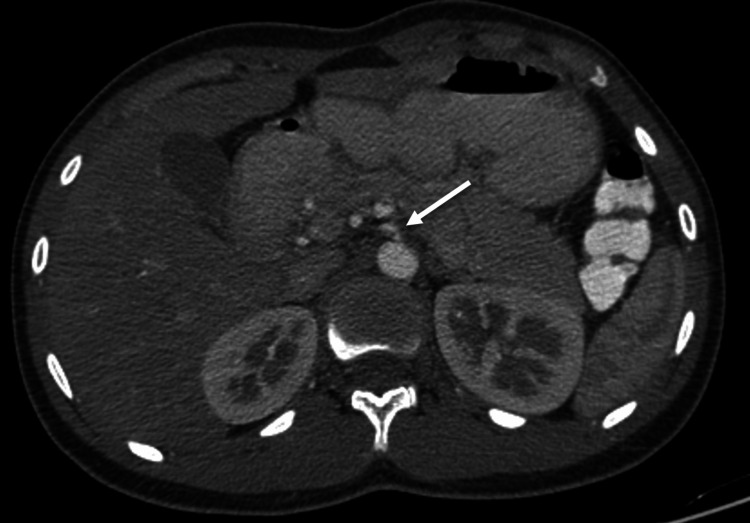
Axial contrast-enhanced CT at the T12–L1 level shows a hypoplastic celiac trunk (arrow) arising from the aorta, demonstrating reduced caliber in cross-sectional view.
